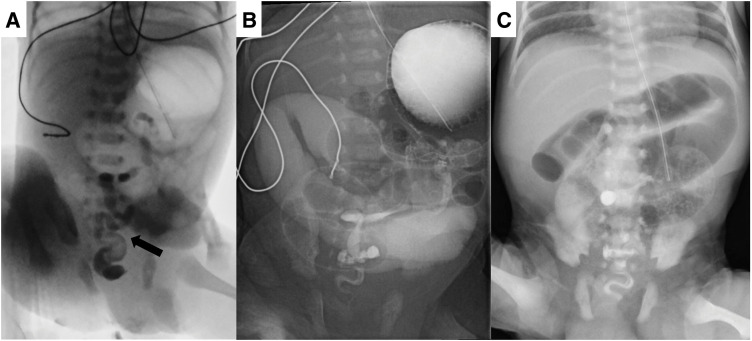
(A) A lower GI examination revealed a small-caliber colon (arrow). No luminal dilatation or transitional zones were observed. (B) An upper GI examination revealed a patent duodenum with dilated small bowel loops. (C) The 1st abdominal X-ray at our hospital revealed dilated bowel loops, suggestive of intestinal obstruction. GI, gastrointestinal
